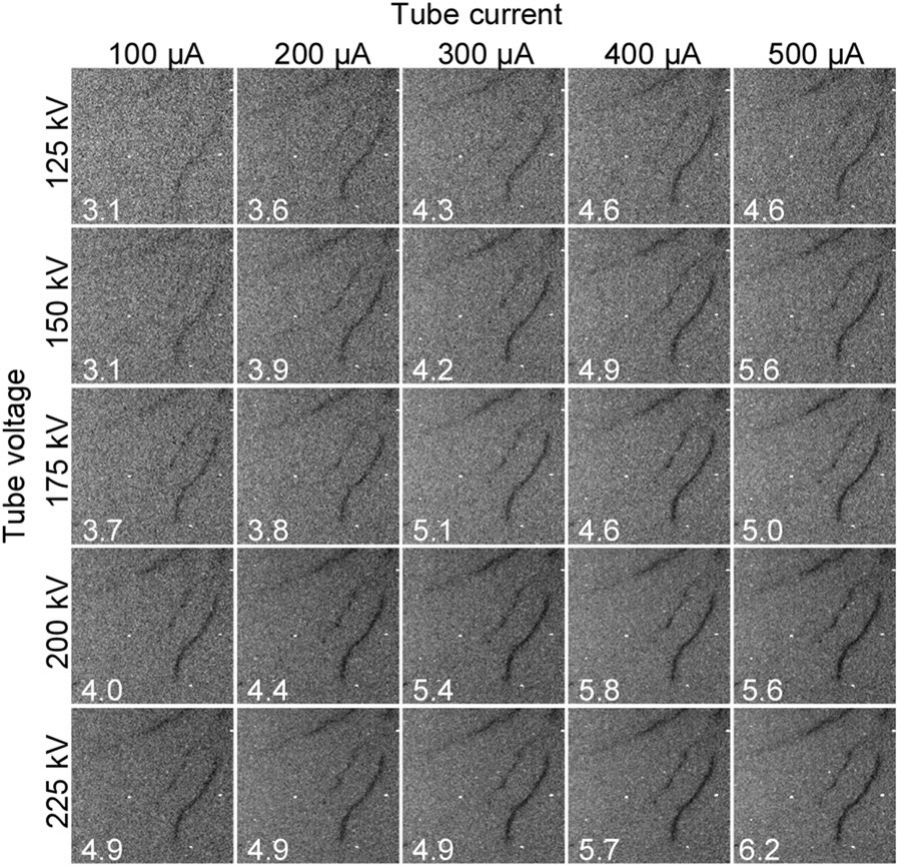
Influence of tube voltage and current on X-ray CT images, using a 4-week-old Kinandang Patong as the scanning subject. Scaled-up vertical slices are shown, using combinations of 125, 150, 175, 200, and 225 kV tube voltages, and 100, 200, 300, 400, and 500 μA tube currents. Numbers on the images indicate the contrast-to-noise ratio
